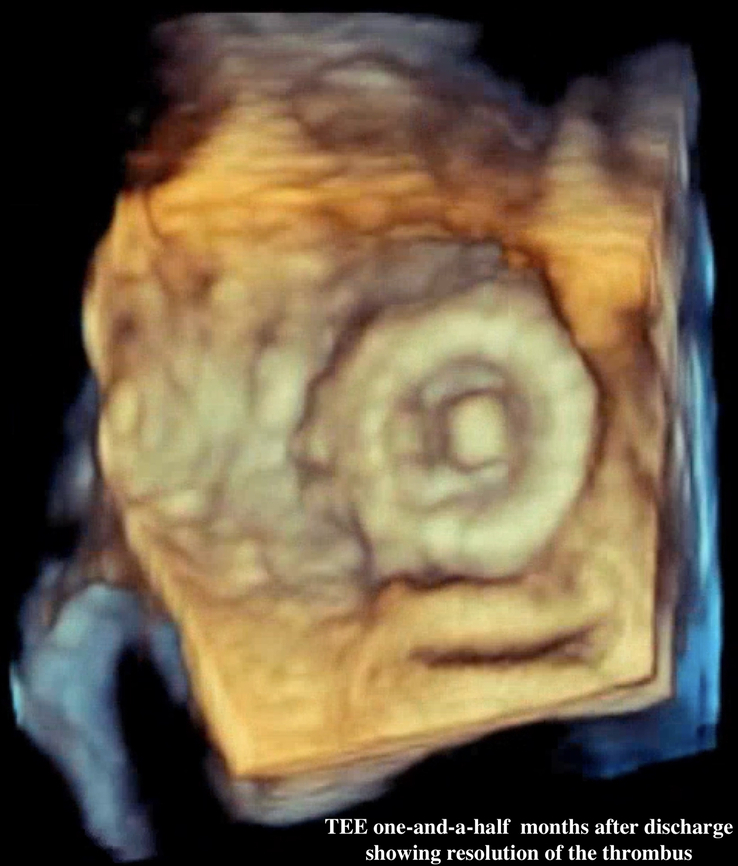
Transesophageal echocardiography image obtained 1.5 months after discharge showing thrombus resolution.
